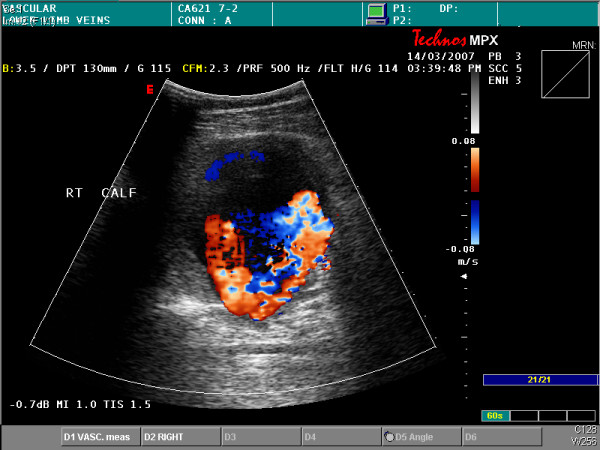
Colour duplex sonography showing a large aneurysm in the posterior tibial artery.
